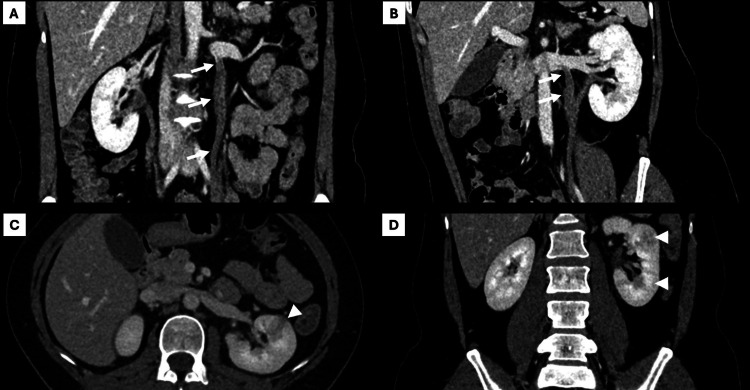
Contrast-enhanced abdominopelvic CT showing left OVT with concurrent ipsilateral acute pyelonephritis (A-B) Coronal multiplanar reconstructions show a dilated left ovarian vein with an intraluminal low-attenuation filling defect, consistent with left OVT (arrows). (C-D) Axial and coronal images show features of acute pyelonephritis, including a striated nephrogram and focal wedge-shaped areas of decreased enhancement in the left kidney (arrowheads) CT: computed tomography; OVT: ovarian vein thrombosis
